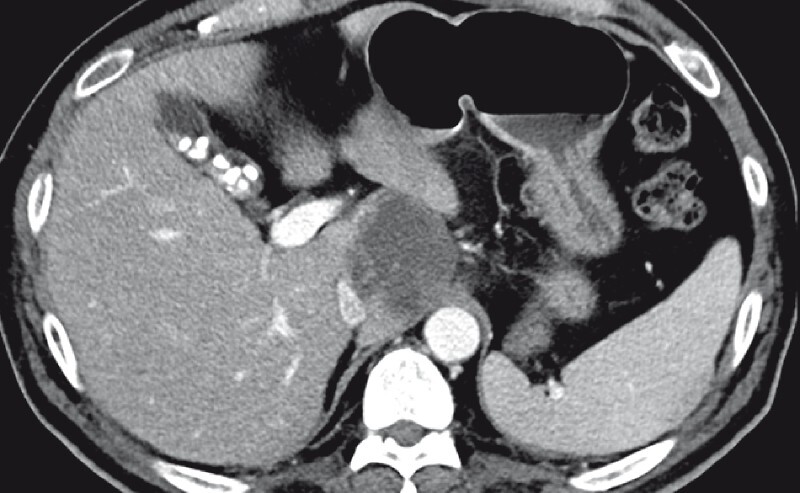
Computed tomography showing a 4.5 × 5.1-cm abscess in the caudate lobe of the liver.
